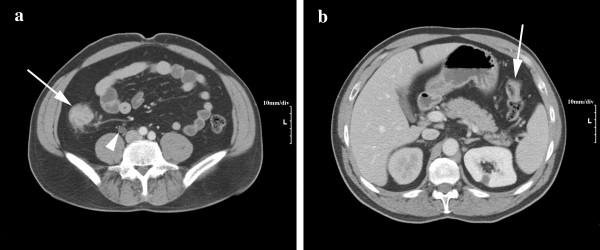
(a) CT scan with PO/IV contrast suggested a large tumor of the ascending colon (arrow). Enlarged pericolic lymph nodes are suggestive of metastases. Also shown is right-sided hydroureteronephrosis caused by the mid-ureteral tumor (arrowhead). (b) The colon tumor at the splenic flexure tumor is visible on a different cut (arrow).
